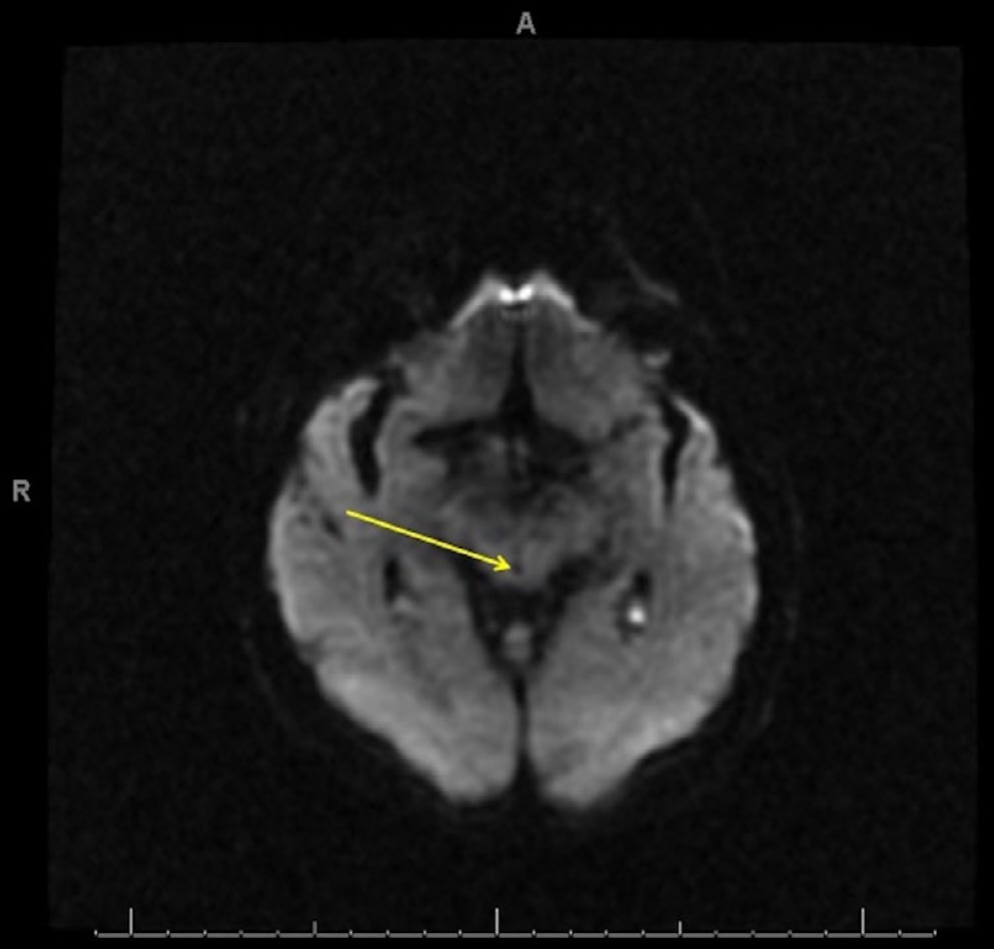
Hospital day 1 axial diffusion‐weighted image (DWI) at the level of the midbrain. No hyperintensities or other abnormal signal intensities surrounding the aqueduct of Sylvius (arrow)
